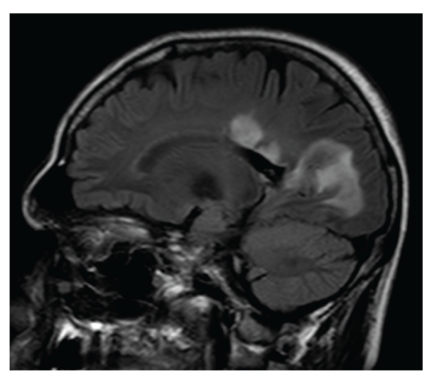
Sagittal FLAIR brain MRI shows a lesion cranial to the corpus callosum and the large occipital lesion.
